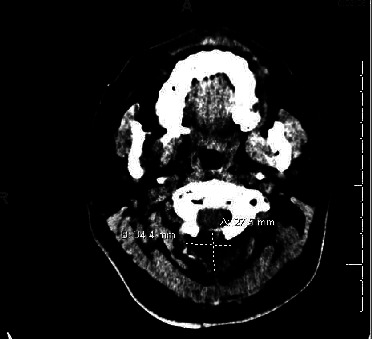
CT imaging (transverse section) showing surgical site collection including a pseudomeningocele and epidural abscess.
